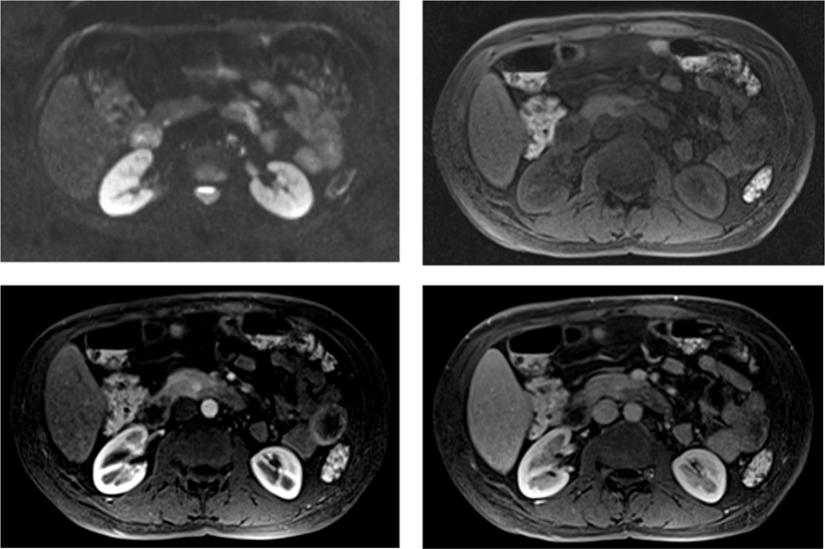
Magnetic resonance images showing the small solid nodule with approximately 1 cm in the pancreatic uncinate process (arrows) with diffusion restriction (upper left image) and hypervascular enhancement (lower left image)
